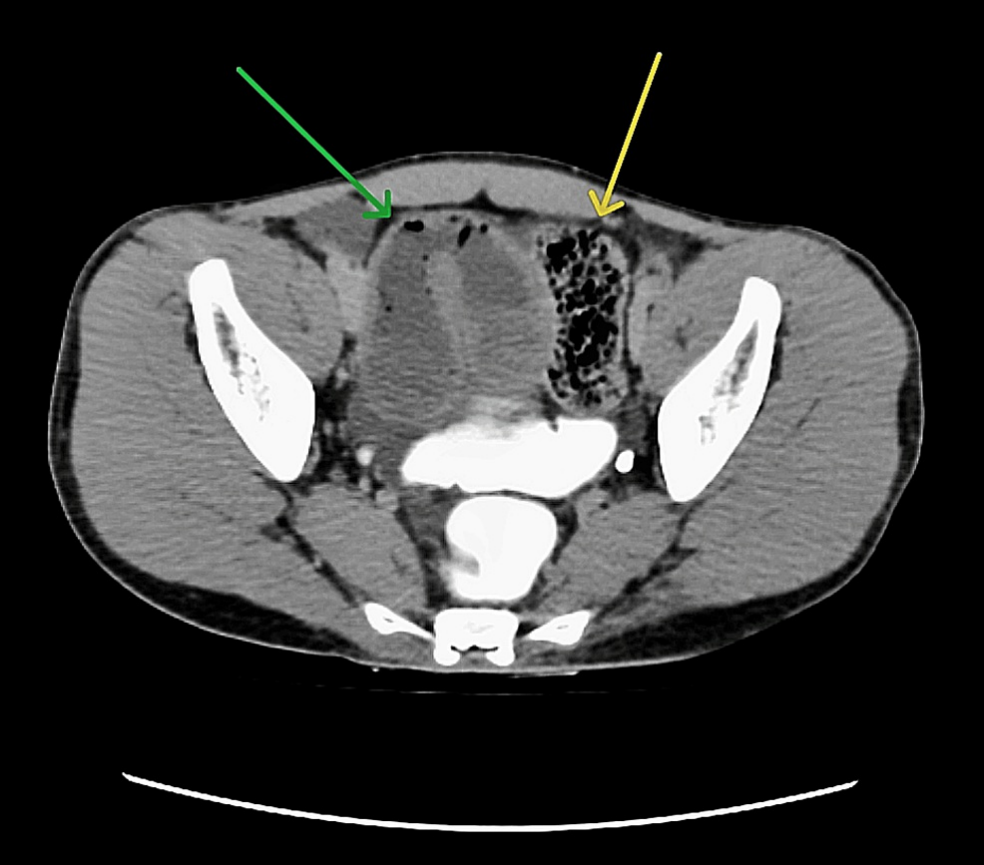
CECT scan of abdomen and pelvis (axial section) CECT shows a closed loop of small bowel in the pelvis (green arrow), with gross dilatation of the involved small bowel loops and thickened, edematous bowel wall. Small bowel feces sign is visible in adjacent bowel loops (yellow arrow), which is the presence of fecal matter intermingled with gas bubbles in the lumen of small bowel, occurring due to reduced intestinal motility and obstruction to the passage of fecal material. These features are suggestive of closed-loop small bowel obstruction. CECT: contrast-enhanced computed tomography
